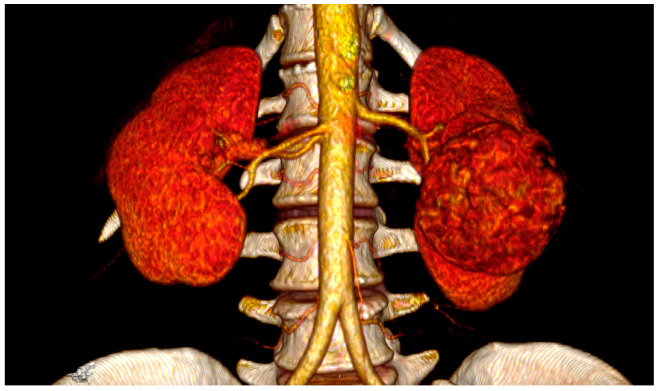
Three-dimensional reconstruction of renal vascular anatomy on axial computed tomography scan with intravenous contrast: a single LRA and double RRA are easily recognizable.
